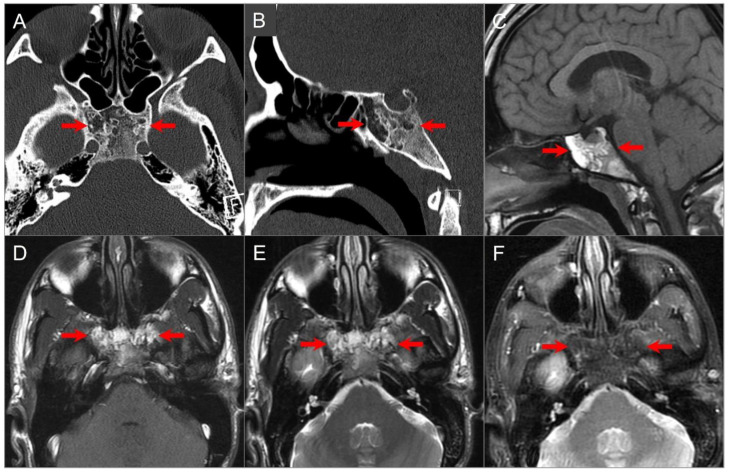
Arrested pneumatization. (A) Axial and (B) sagittal CT images show partial pneumatization of the sphenoid sinuses anteriorly with a heterogeneous appearance of the sphenoid body. There are multiple hypodense foci with peripheral sclerosis, a non-expansile and non-destructive appearance, and similar density to subcutaneous fat (red arrows). (C) Sagittal and (D) axial noncontrast T1W and (E) axial T2W images reveal heterogeneous hyperintense signals in these regions. (F) Fat-suppressed T2W image shows signal suppression consistent with fatty bone marrow.
